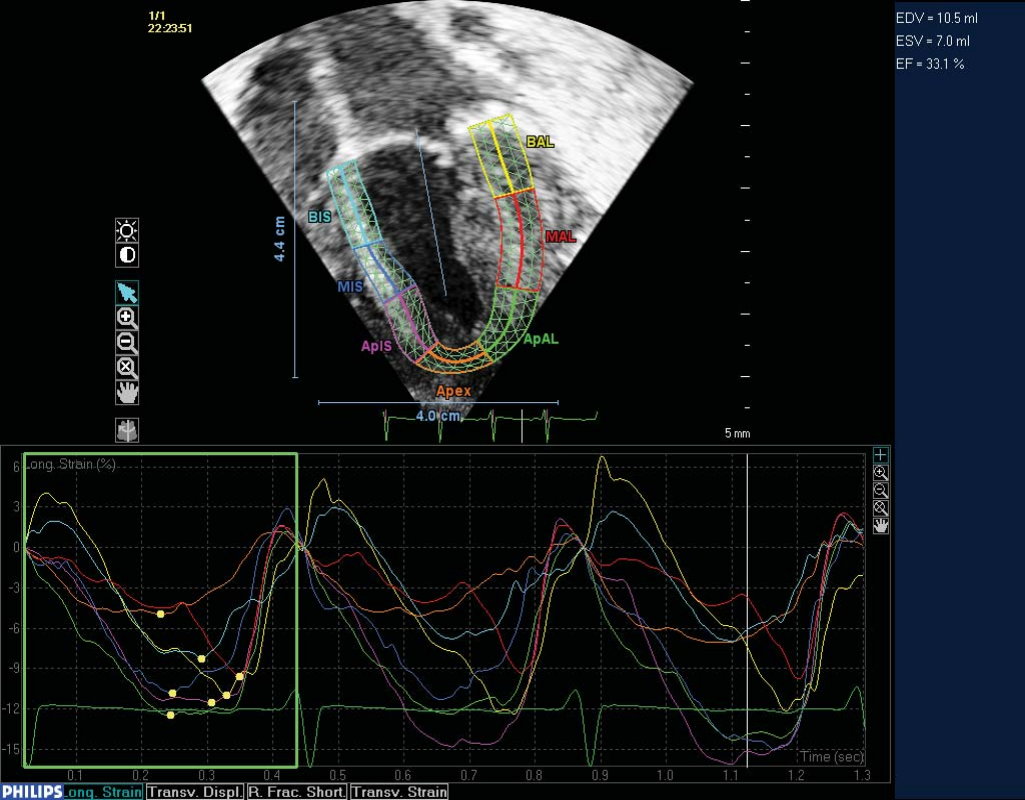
Apical four chamber view of the left ventricle in a neonate post surgical aortic valvotomy. Two dimensional speckle tracking demonstrating
